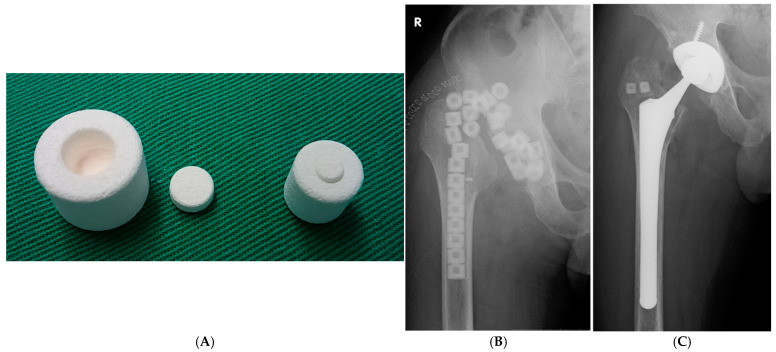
(A): Photograph of a calcium hydroxyapatite (CHA) ceramic block. (B): Radiographs of the right hip of a 58-year-old man (Case 9). Anteroposterior radiograph after the removal of all components and insertion of antibiotic-impregnated CHA into the bone and joint space. (C): Anteroposterior X-ray image after revision total hip arthroplasty, showing CHA blocks implanted in the acetabulum and major trochanter.
